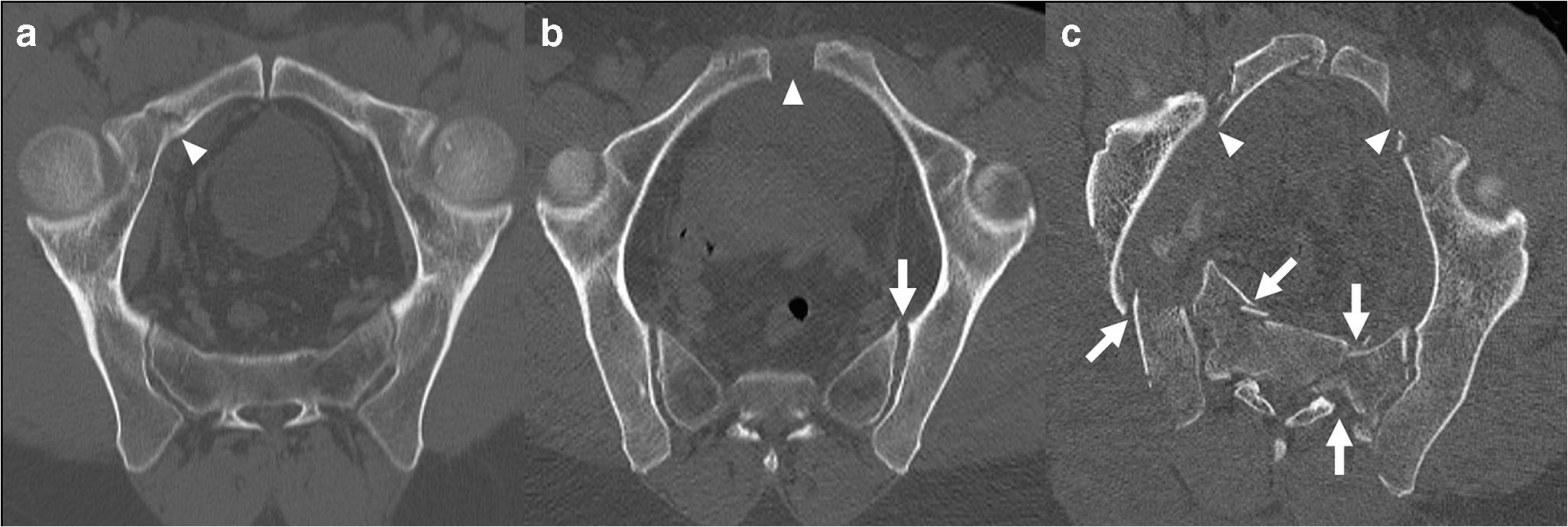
Representative axial-oblique reformatted CT images showing the pelvic ring of patients with Tile A (2.2) (a), Tile B (1.1) (b), and Tile C (3.3) (c) fracture types. Arrowheads show disruption of the anterior arch, while arrows indicate disruption of the posterior arch of the pelvic ring. In Tile A fractures, the posterior arch is spared, while it is partially disrupted in Tile B and completely disrupted in Tile C fracture types
